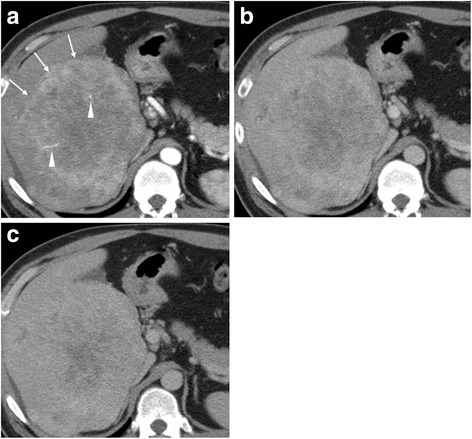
Computed tomography images. a Arterial-phase scan shows a peripherally well-enhanced tumor in the right liver (*arrow*) with vessel penetration (*arrow head*). Retention of the contrast media in the lesion is observed b from portal phase c to delayed phase
